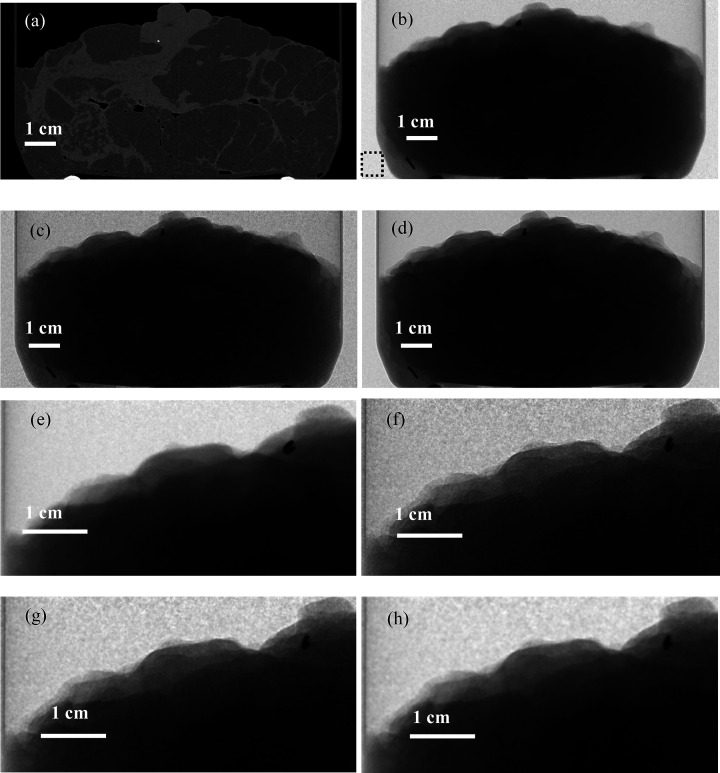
Images of a mastectomy sample collected using plane monochromatic X-rays with an energy of 32 keV. (*a*) Reconstructed axial CT slice through the middle of the sample. (*b*) PBI projection at the sample-to-detector distance of 19 cm and 3.33 µGy dose. The dashed square in the lower left corner outlines the region inside which the SNR measurements were performed in all the images. (*c*) PBI projection at the sample-to-detector distance of 600 cm and 0.67 µGy dose. (*d*) PBI projection at the sample-to-detector distance of 600 cm and 4 µGy dose. (*e*) Zoomed sub-image of (*b*). (*f*) Zoomed sub-image of (*c*). (*g*) Zoomed sub-image of the TIE-Hom reconstruction with γ = 275 from the image shown in (*c*). (*h*) Zoomed sub-image of the TIE-Hom reconstruction with γ = 869 from the image shown (*c*).
